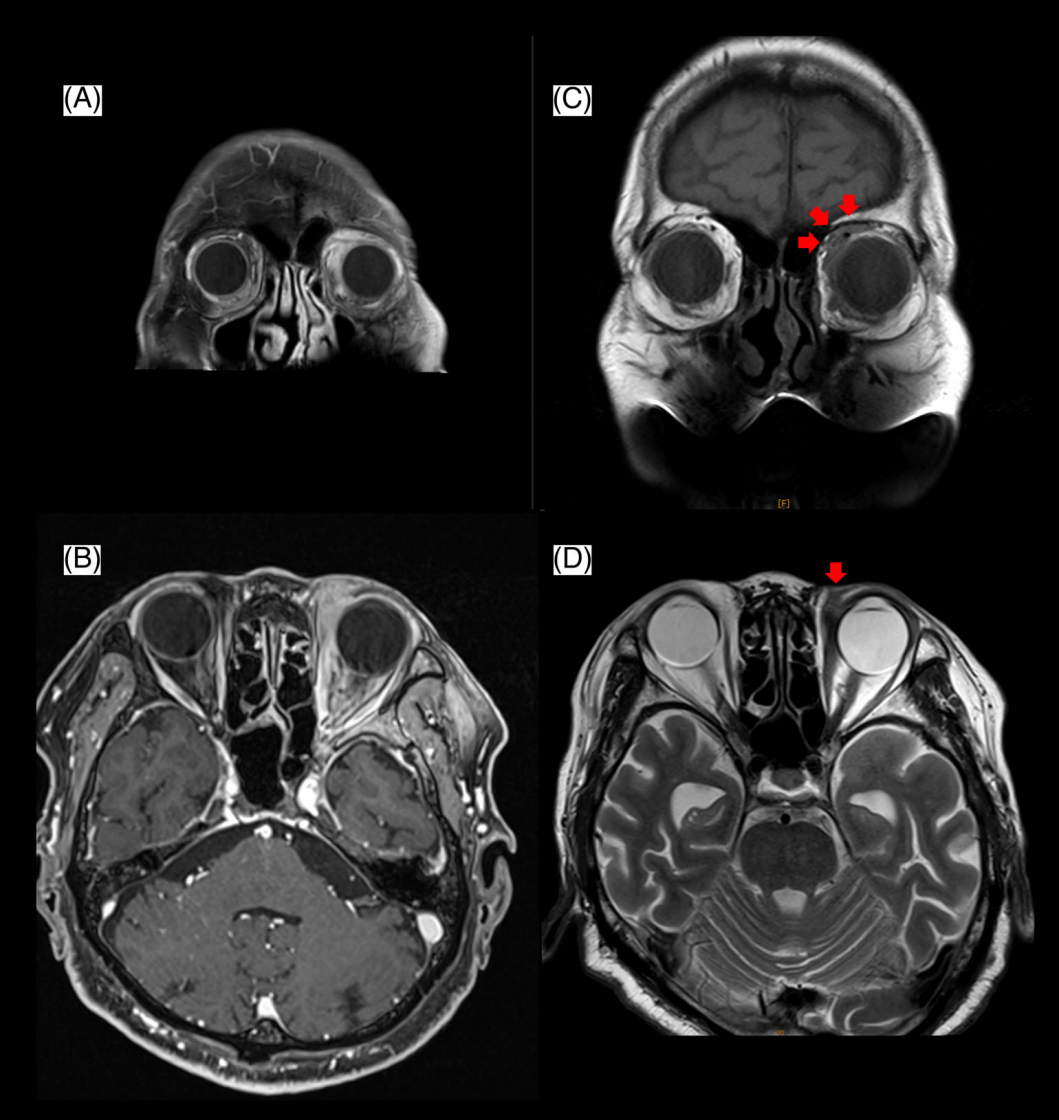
Magnetic resonance imaging (MRI) finding of orbital extranodal marginal zone lymphoma (EMZL) 1 month after concurrent chemoradiotherapy for lung cancer. (A, B) Orbital EMZL at diagnosis on enhanced MRI. (C, D) Orbital mass EMZL 1 month after chemotherapy on plain MRI.
